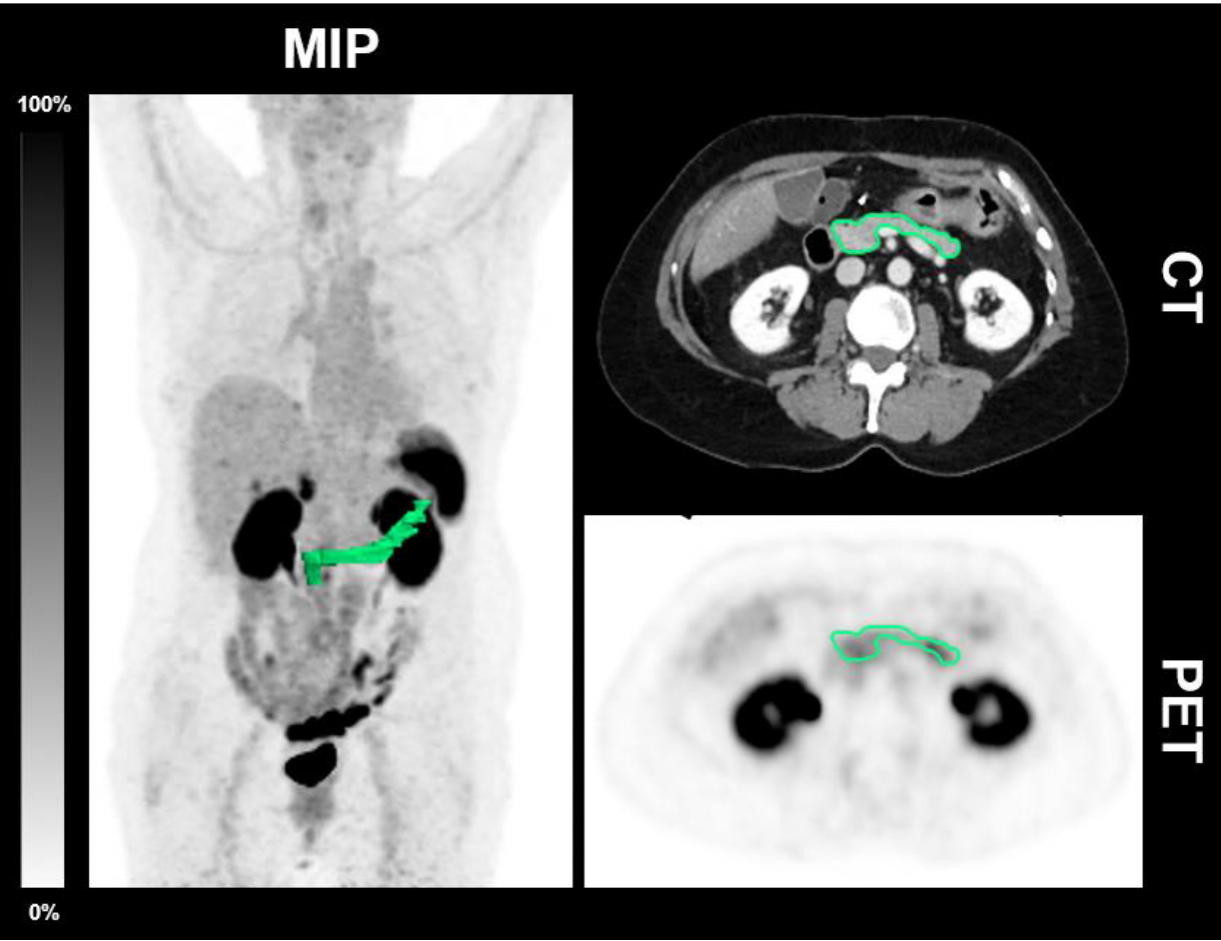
CT-derived delineation of the pancreas in a patient without T2D. Maximum intensity projection (MIP), axial CT and PET of a 57-year-old female patient diagnosed without T2D (HbA_1c_ 5.6%; 38 mmol/l) undergoing [^68^Ga]Ga-DOTATOC-PET/CT demonstrating the CT morphological delineation of the pancreas. The SUV_max_ was 5.0 and the SUV_mean_ 2.5.
